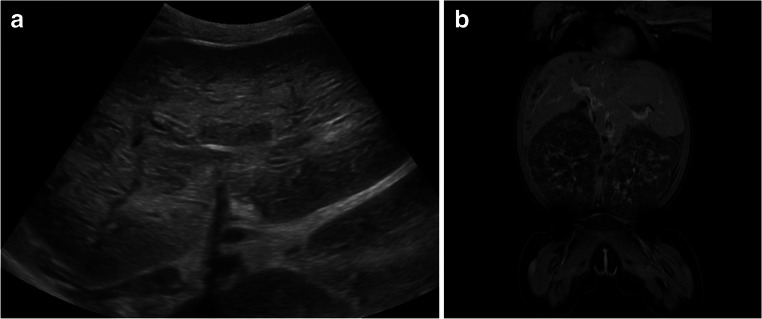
Radiological presentation of ARPKD with enlarged kidneys detected by ultrasound (a) and magnetic resonance imaging (b)
